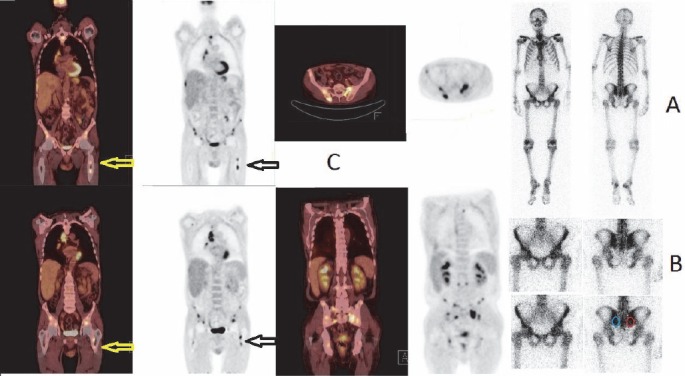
Bone marrow involvement according to ^18^F-FDG PET/CT scan and whole body bone scintigraphy 6 days after ^153^Sm-EDTMP bone pain palliation therapy
